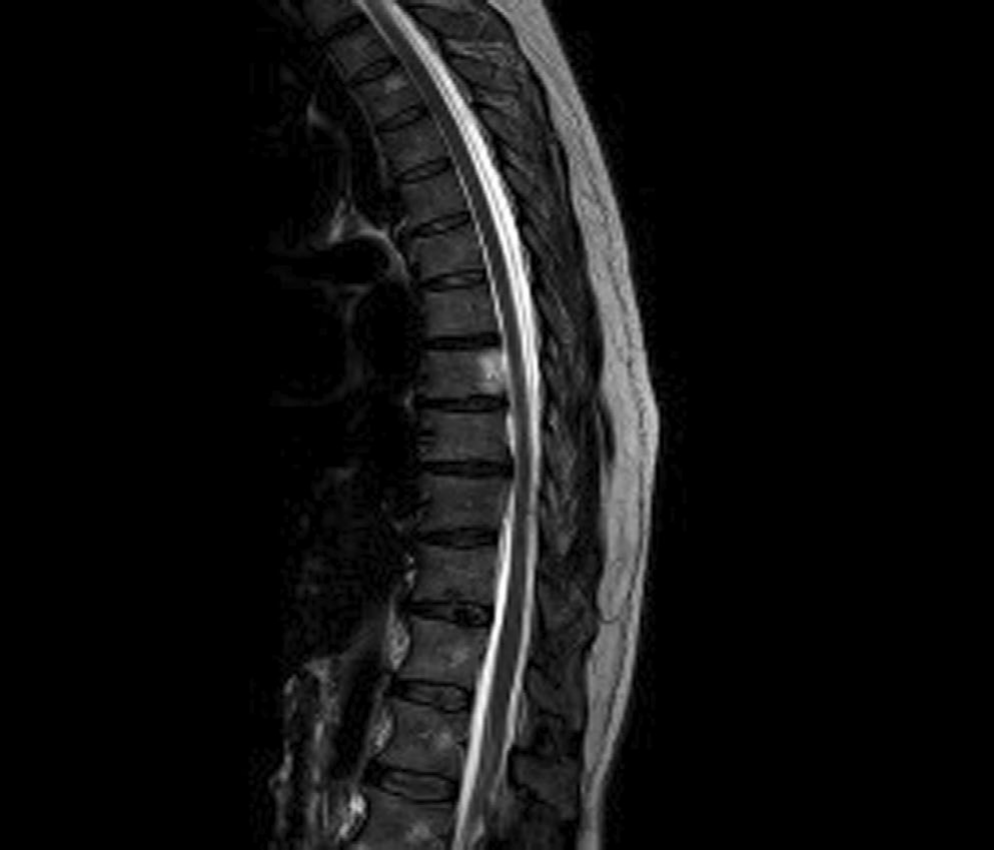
FIGURE 1 Dural detachment in sagital spinal cord MRI
